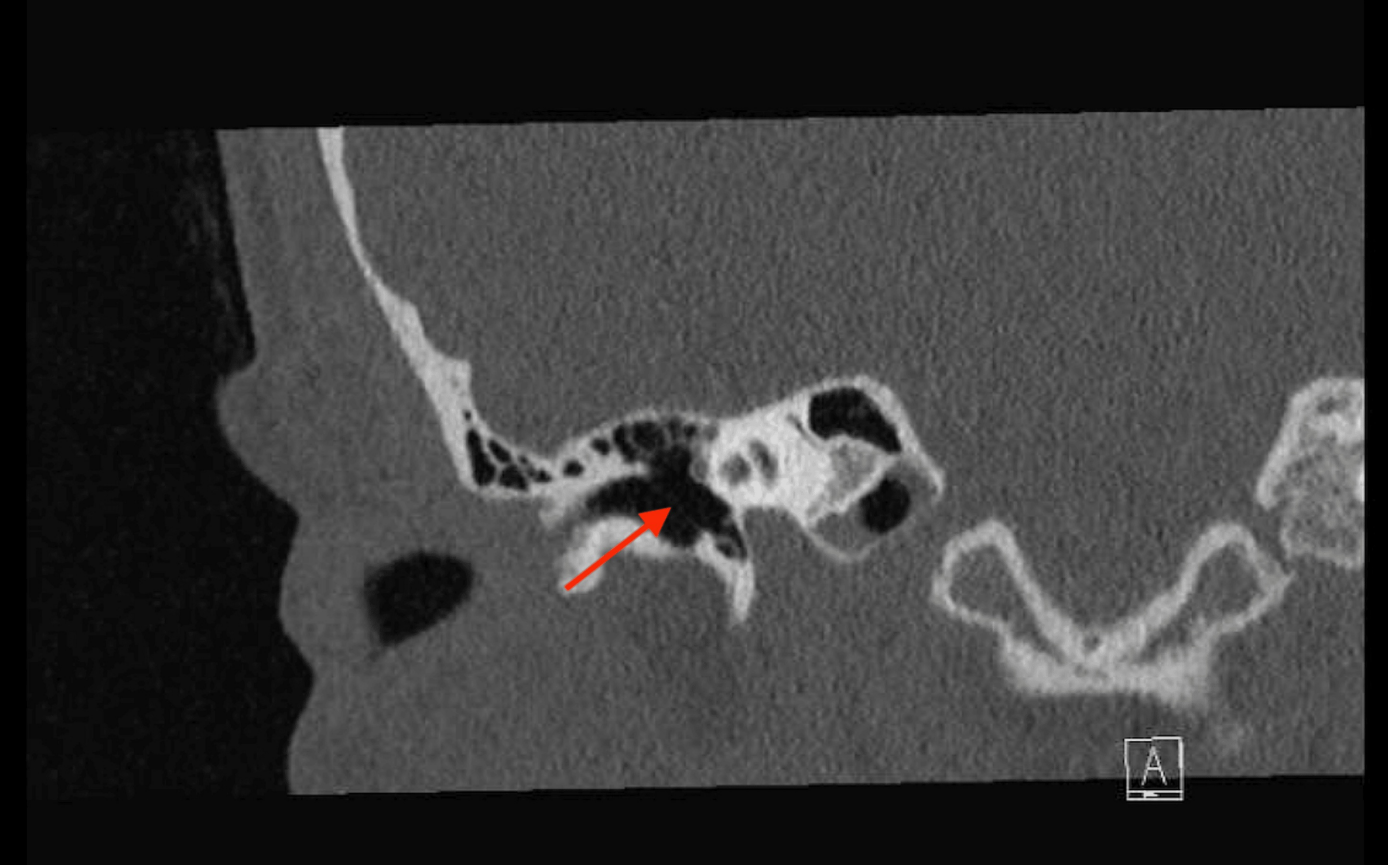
Coronal view of a temporal CT scan without contrast, showing the right internal acoustic canal with a normal right tympanic membrane (indicated by a red arrow)
